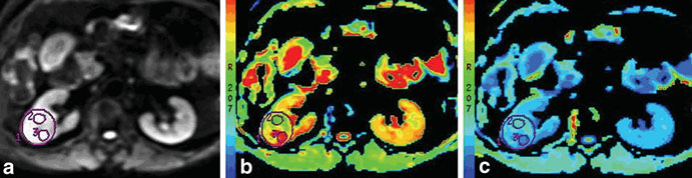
DWI map, EADC map and ADC map showing the parenchymal part and cystic part of tumor at multiple levels. a DWI map: the overall ROI1 of tumor appears as mixed signals, with diffusion-restricted high signals in parenchymal part (ROI3) and equal signals in cystic part (ROI2); b EADC map: ROI1 0.134, ROI2 0.0752, ROI3 0.256; c ADC map ROI1 2.102 × 10^−3^ mm^2^/s, ROI2 2.601 × 10^−3^ mm^2^/s, ROI3 1.372 × 10^−3^ mm^2^/s
